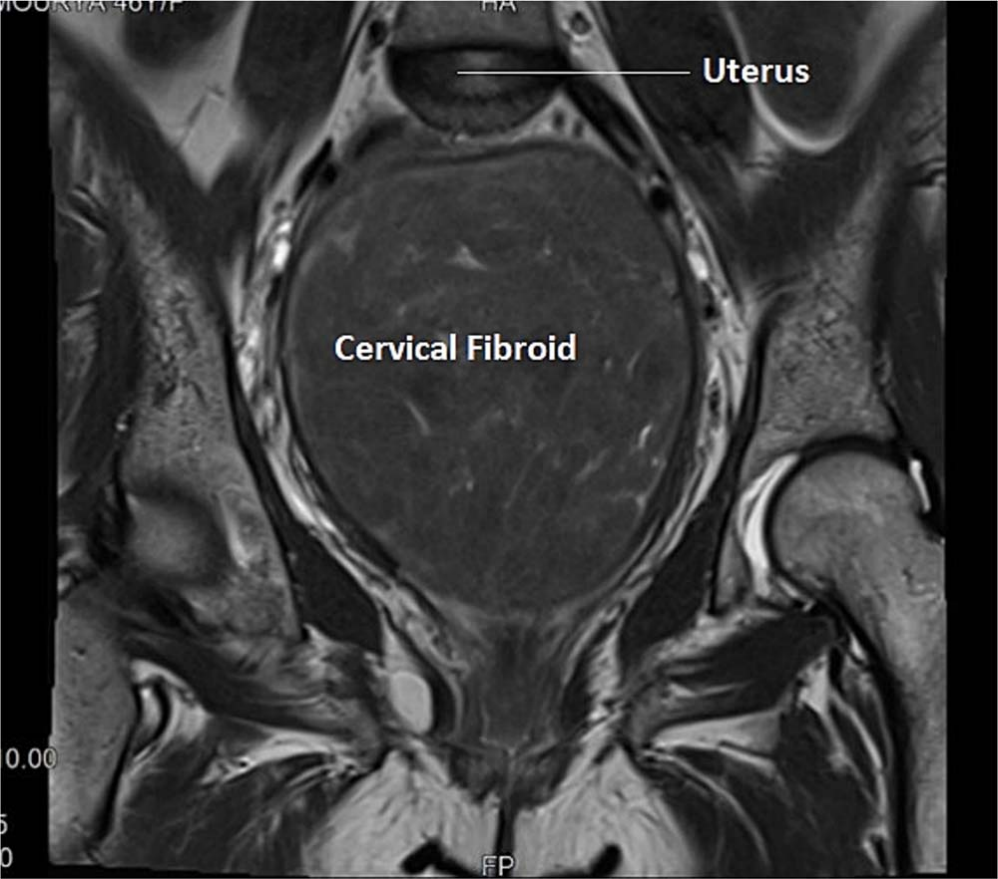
Transverse section of MRI of Case 2 – similar multi-sequential transverse sections aids in clear delineation of course of ureter and its relationship with uterine artery in this case of distorted anatomy because of giant cervical fibroid.
